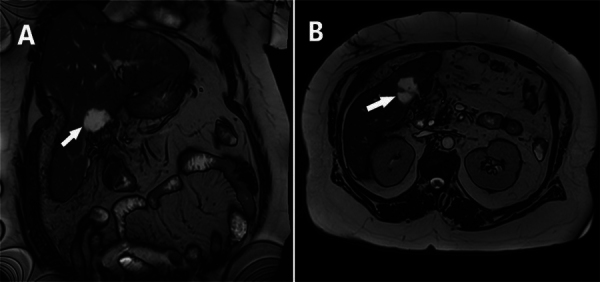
Coronal (A) and axial (B) T2-weighted MRI images demonstrate a loculated collection (arrow) in the gallbladder bed.
